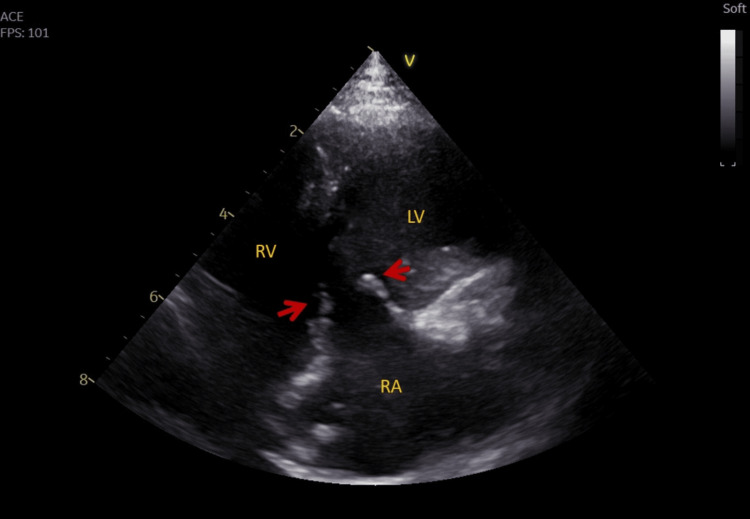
Transthoracic echocardiogram, modified parasternal long axis to see the tricuspid valve Red arrow: tricuspid valve leaflets RA: right atrium; RV: right ventricle; LV: left ventricle
